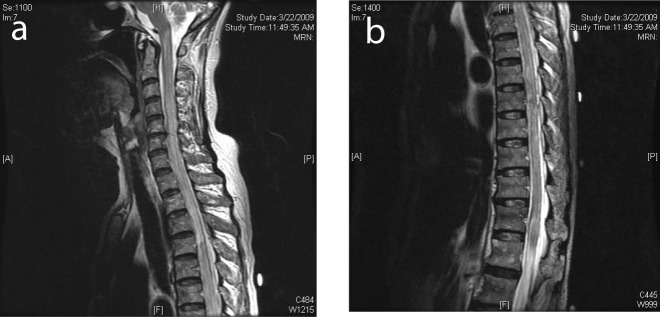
(a) MRI of the spine showing cord edema progressing cranially to involve the cervical spine. (b) MRI demonstrated edema involving the conus medullaris.
